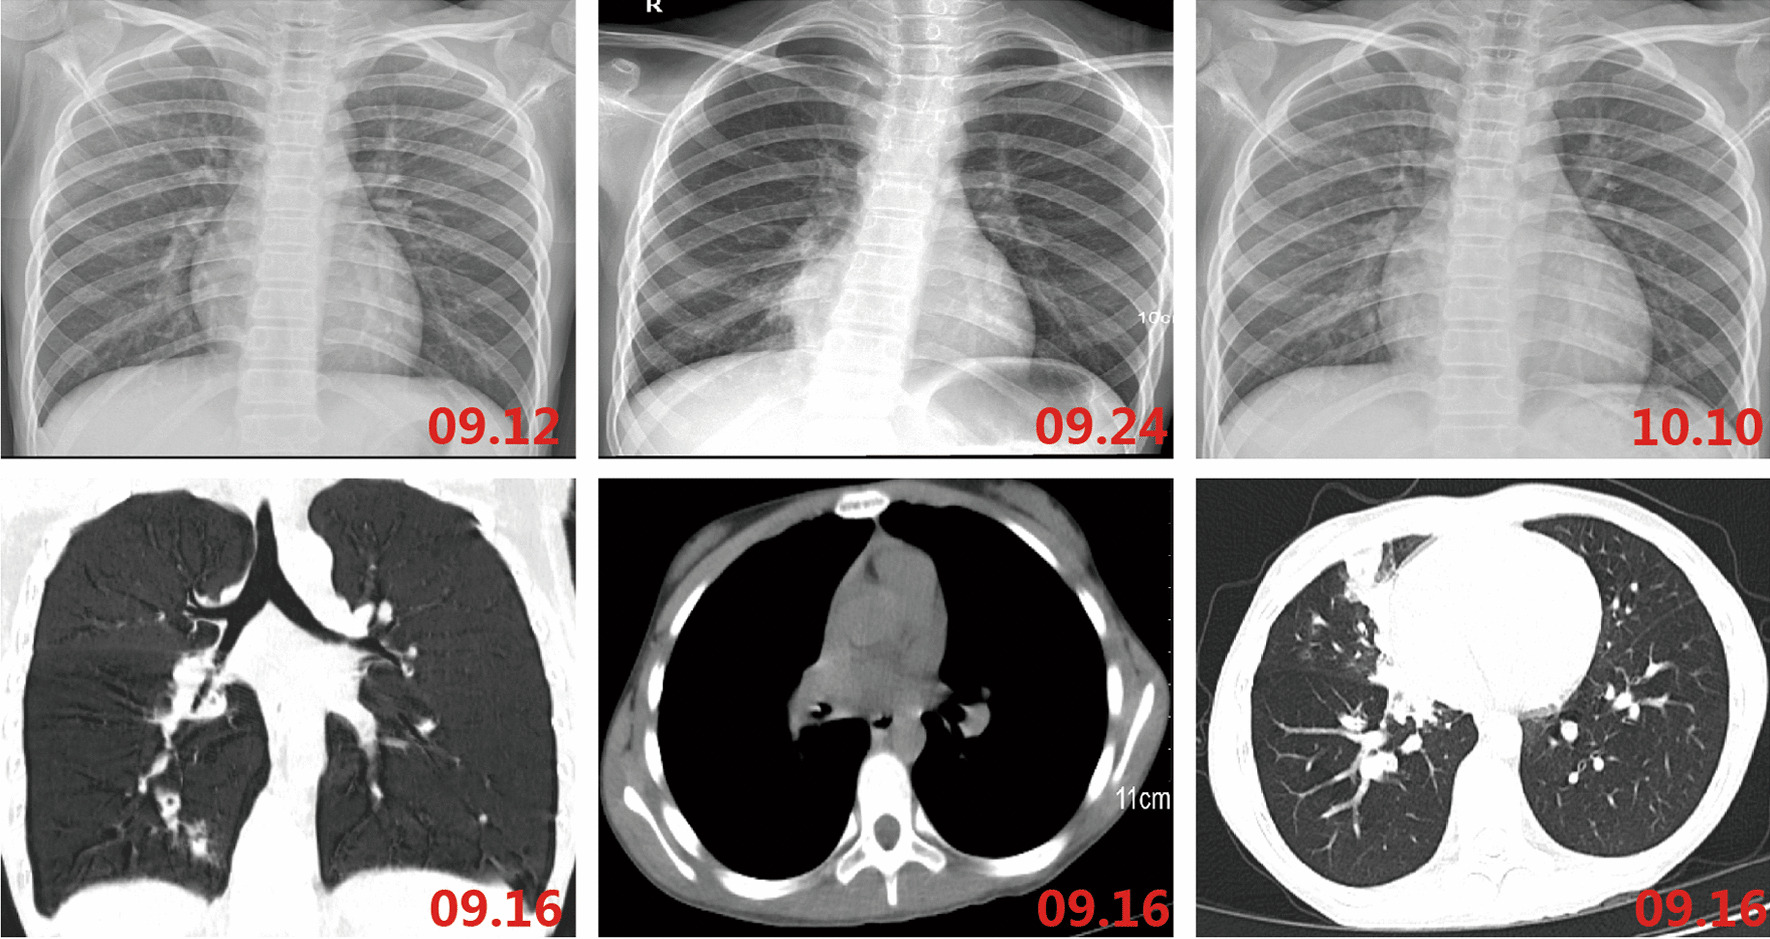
The chest imaging of our case. Chest radiograph showed a fuzzy patchy shadow on the right lower lung. HRCT revealed inflammation of the middle and lower lobe of the right lung. Follow-up chest radiograph prompted basically normal
